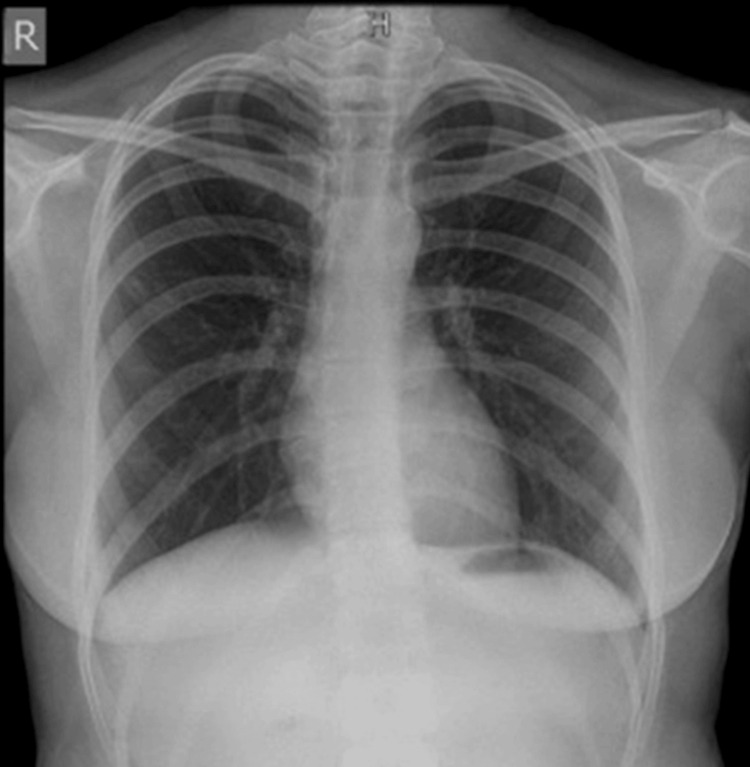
Chest X-ray (PA view) at the completion of antitubercular therapy demonstrating complete resolution of right-sided pleural effusion Follow-up radiograph confirming full radiological resolution with clearance of the pleural opacity and restoration of normal lung markings, correlating with the patient's clinical improvement. PA: posteroanterior
